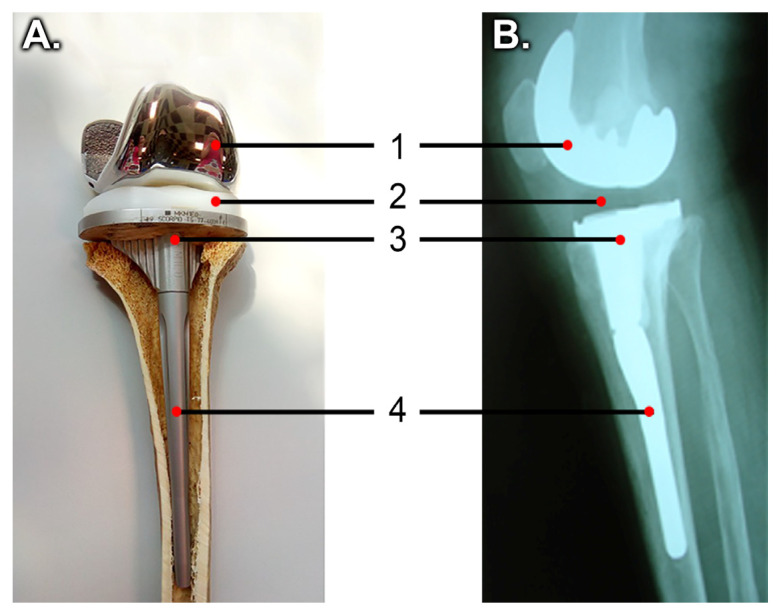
Knee arthroplasty: (A) Tibial bone with prosthesis, laboratory model, (B) Postoperative radiograph ((1) femoral metal implant, (2) polyethylene spacer, (3) tibial metal implant, and (4) tibial stem extension).
